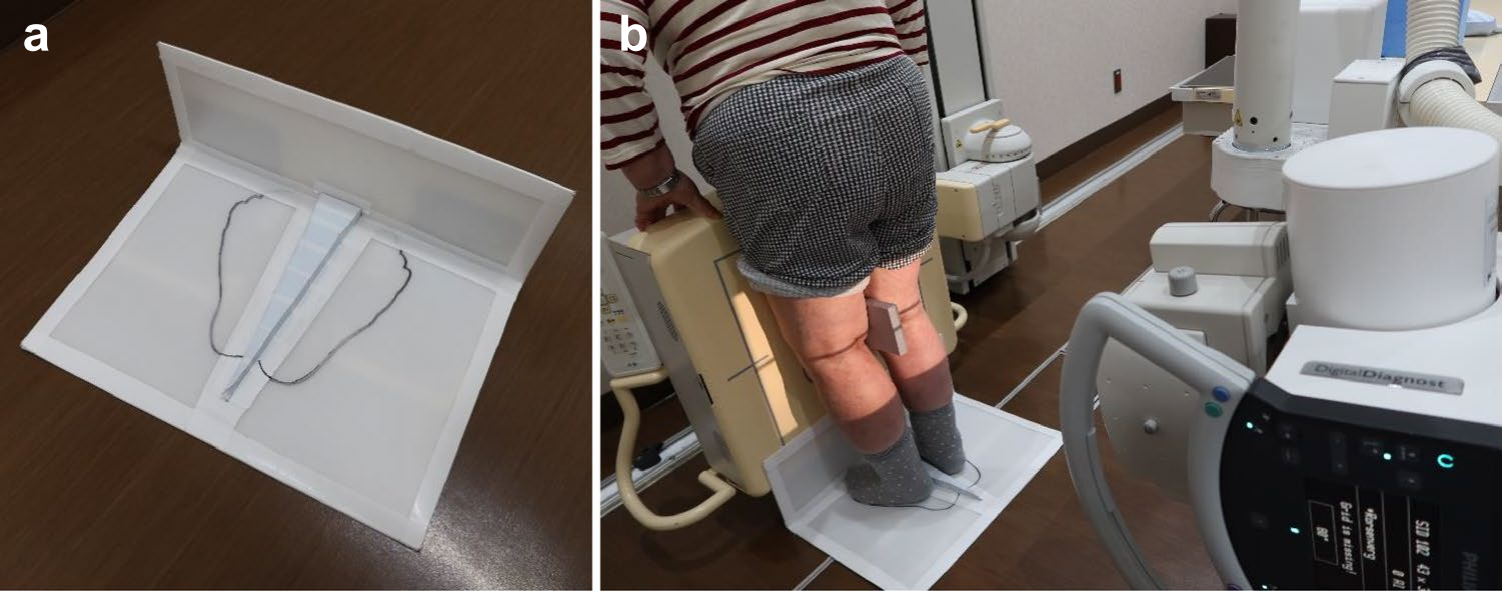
FFV methods. (a) The custom-made limb positioning plate. (b) The patient standing on the custom-made plate while keeping the toes and the front of the thighs in contact with the film cassette. A posteroanterior image is acquired with the X-ray beam angled caudally at 10°. FFV, fixed-flexion view
